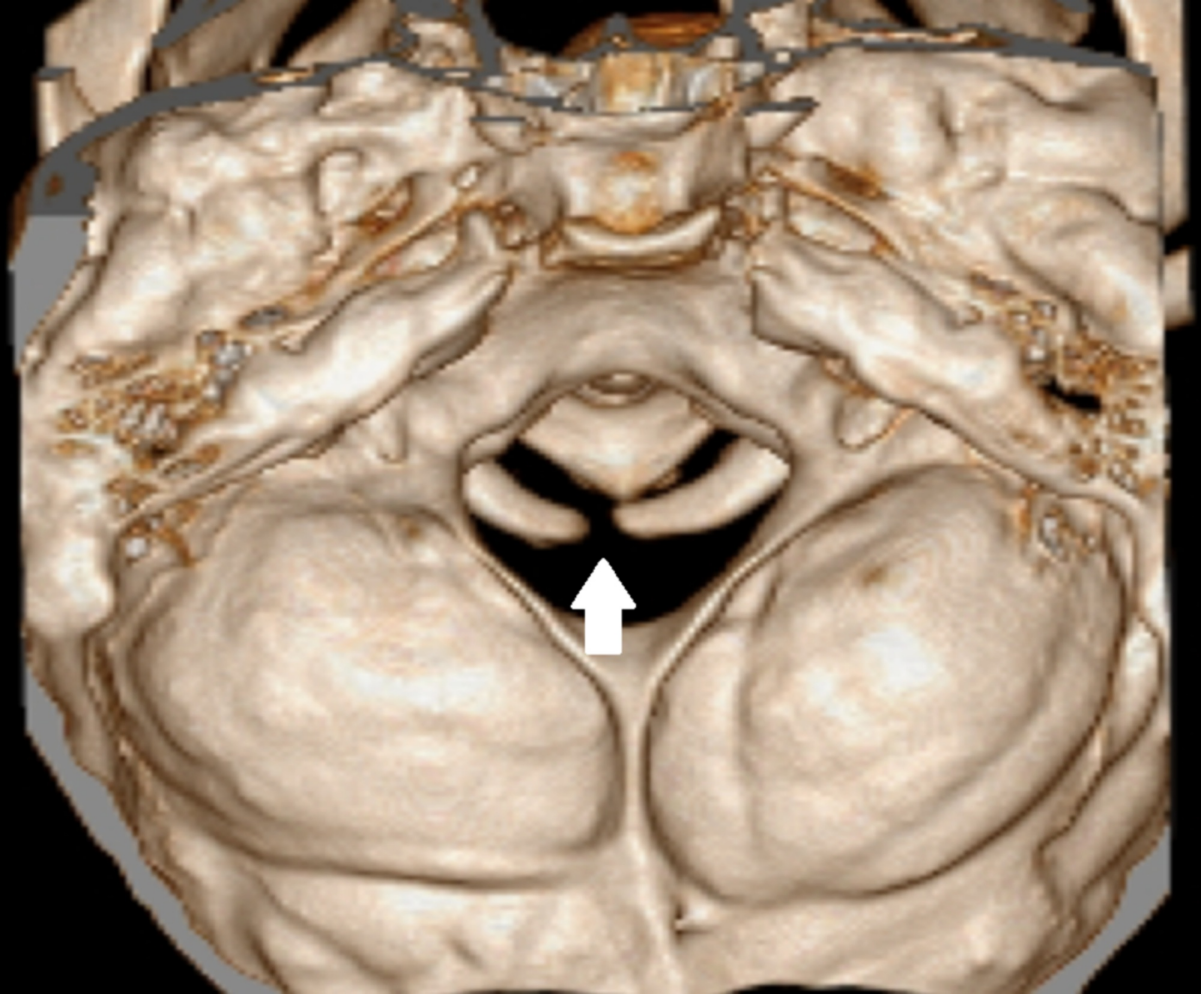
Atlas’ posterior arch defect Three-dimensional CT showing a posterior arch defect of the atlas (white arrow).
